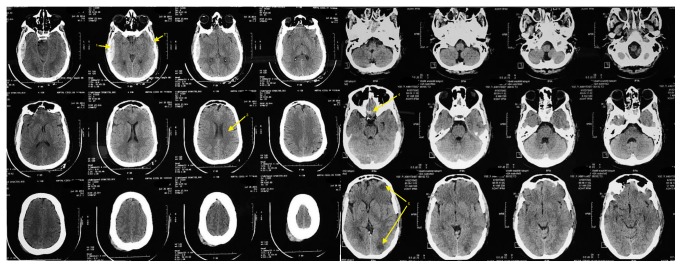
Computed tomography of patient at admission. (1) Extra-dural hematoma of 15 mm. (2) Pneumocephalus. (3) Cerebral edema. (4) Contusions of frontal brain lobe. (5) Extensive ischemia of the entire right hemisphere.
